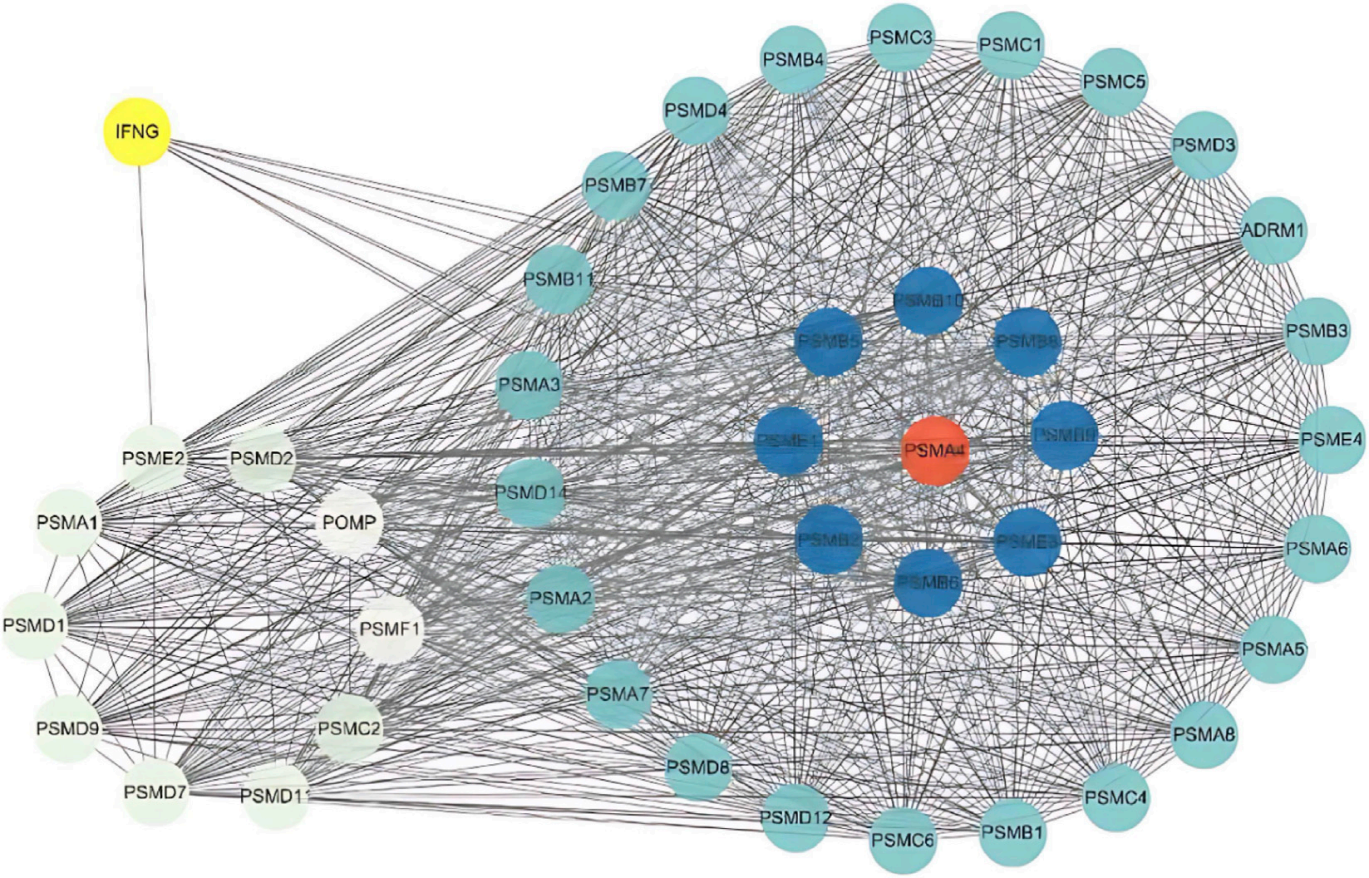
PPI subnetwork for the Proteasome pathway. Interaction subnetwork of proteins involved in the Proteasome pathway, highlighting key proteins like PSMA4 and IFNG.
